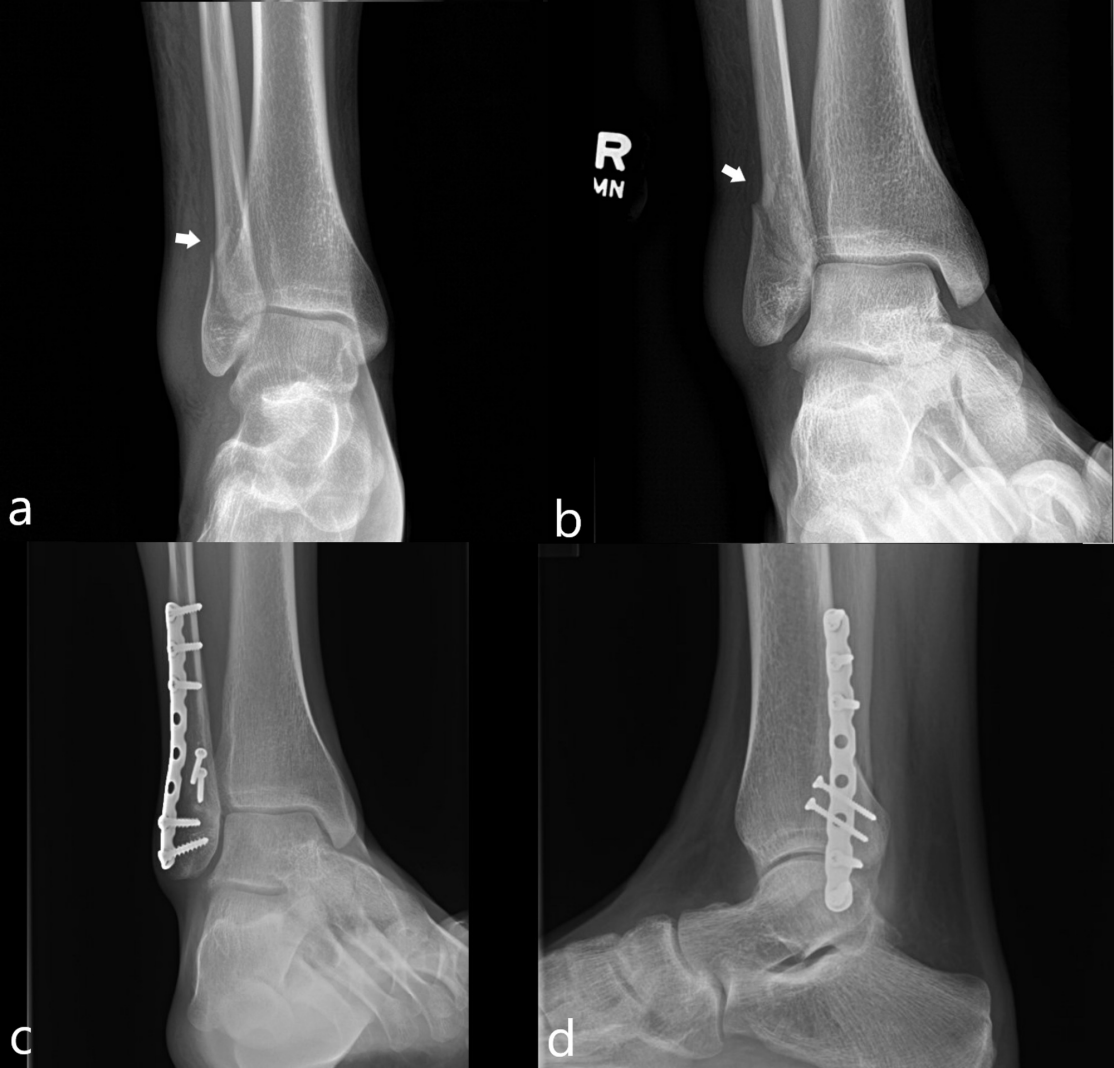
Case 7 radiographic images of the ankle A frontal radiograph of the ankle (a) demonstrates a mildly comminuted fracture of the lateral malleolus(white arrow). An oblique mortise view radiograph of the ankle (b) demonstrates the fracture to a better extent (white arrow). Oblique mortise view (c) and lateral (d) radiographs demonstrate changes related to internal fixation.
